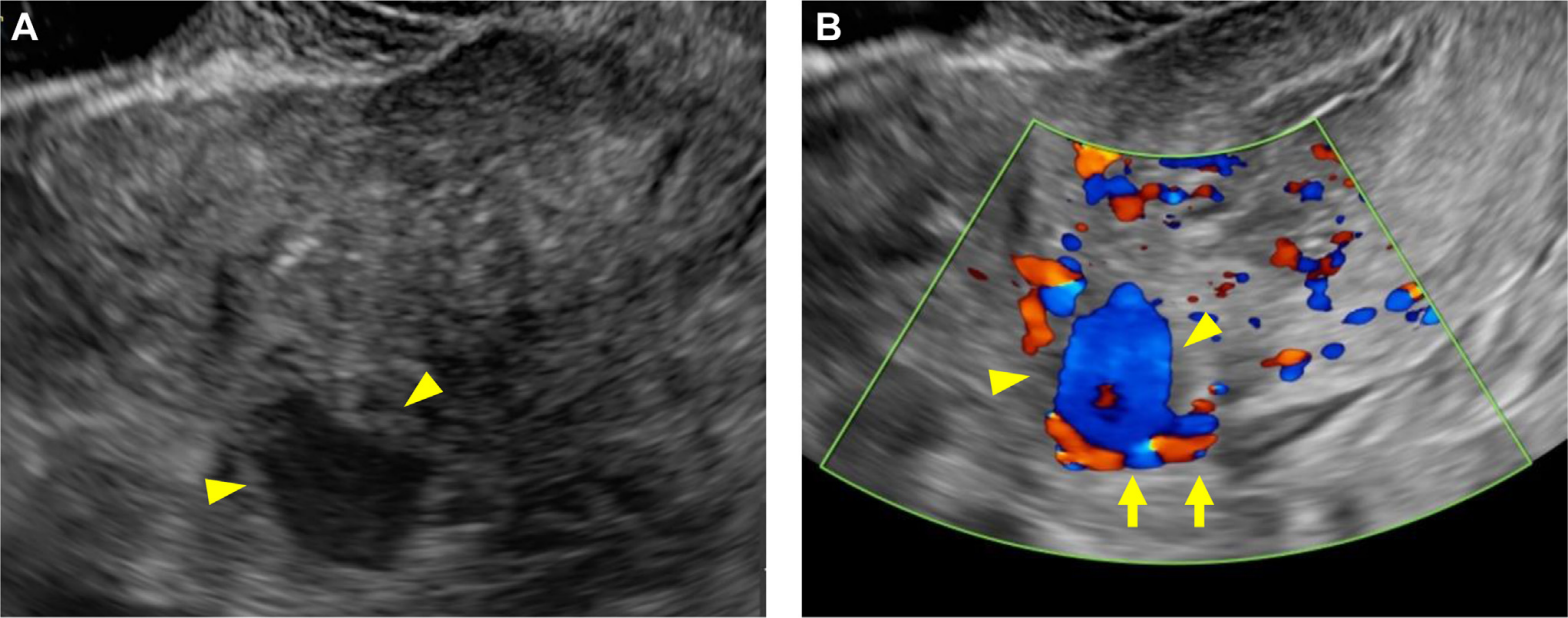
Transvaginal ultrasonography: (A) An 18.6-mm cystic mass (arrowheads) in the left uterine cornu; (B) A mass (arrowheads) with internal blood flow suggestive of hemorrhage (arrows) in the left uterine cornu.
